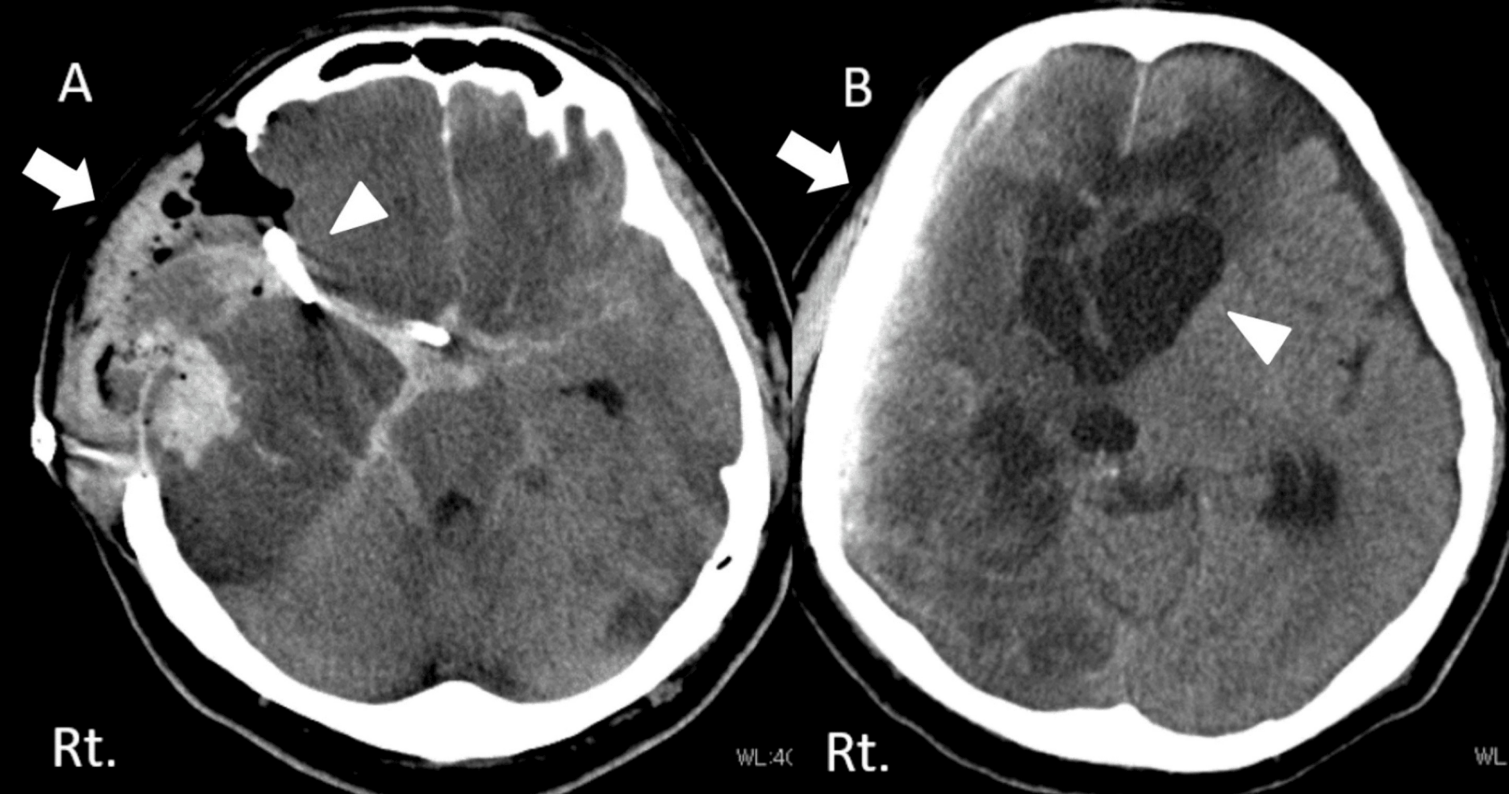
Postoperative CT scan post clipping (A) and post-cranioplasty (B) A: Emergency external decompression surgery was performed with craniotomy clipping (white arrow) and hematoma removal (white arrow) on the right side. B: Head CT scan of the head shows artificial bone cranioplasty (white arrow) and enlargement of the anterior horns (white arrowhead), suggestive of hydrocephalus.
